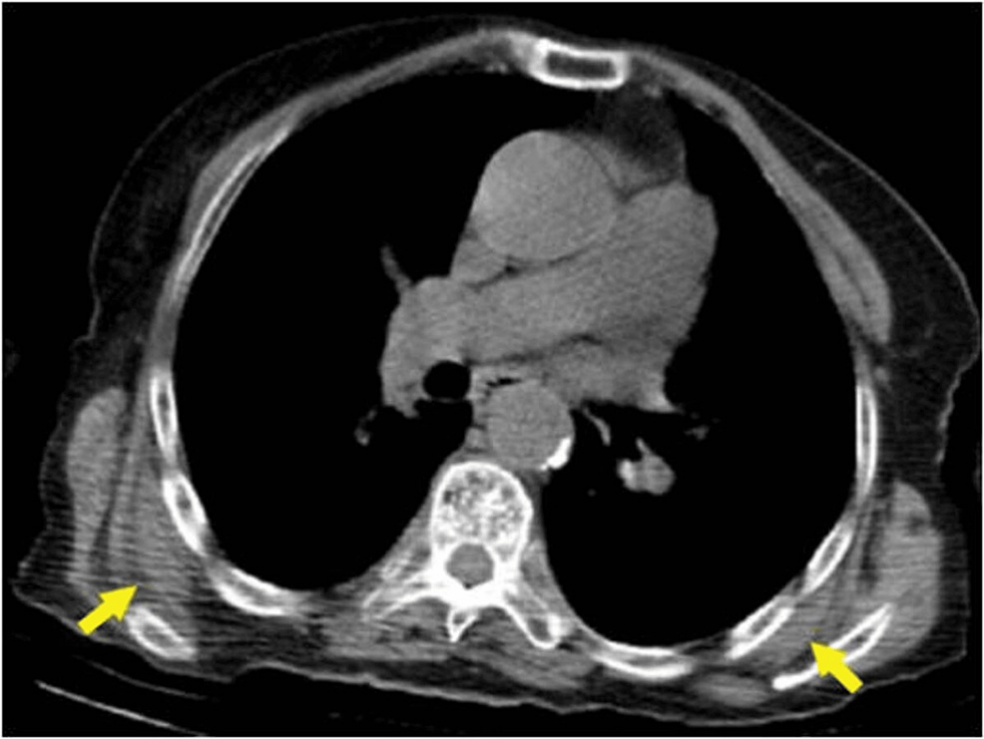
Axial non-contrast CT image of a 85-year-old male patient showing (yellow arrows) bilateral ED; soft tissue located at the posterior thoracic wall, well-circumscribed and isodense with the adjacent muscle structure.
